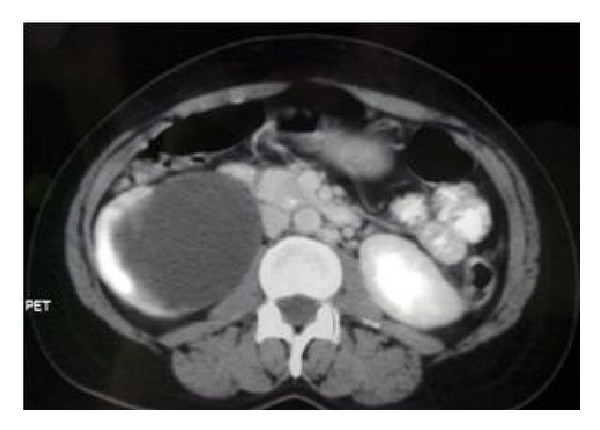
PET CT axial cut showing gross hydronephrosis of right kidney with thinned out parenchyma and left mild hydronephrosis.
